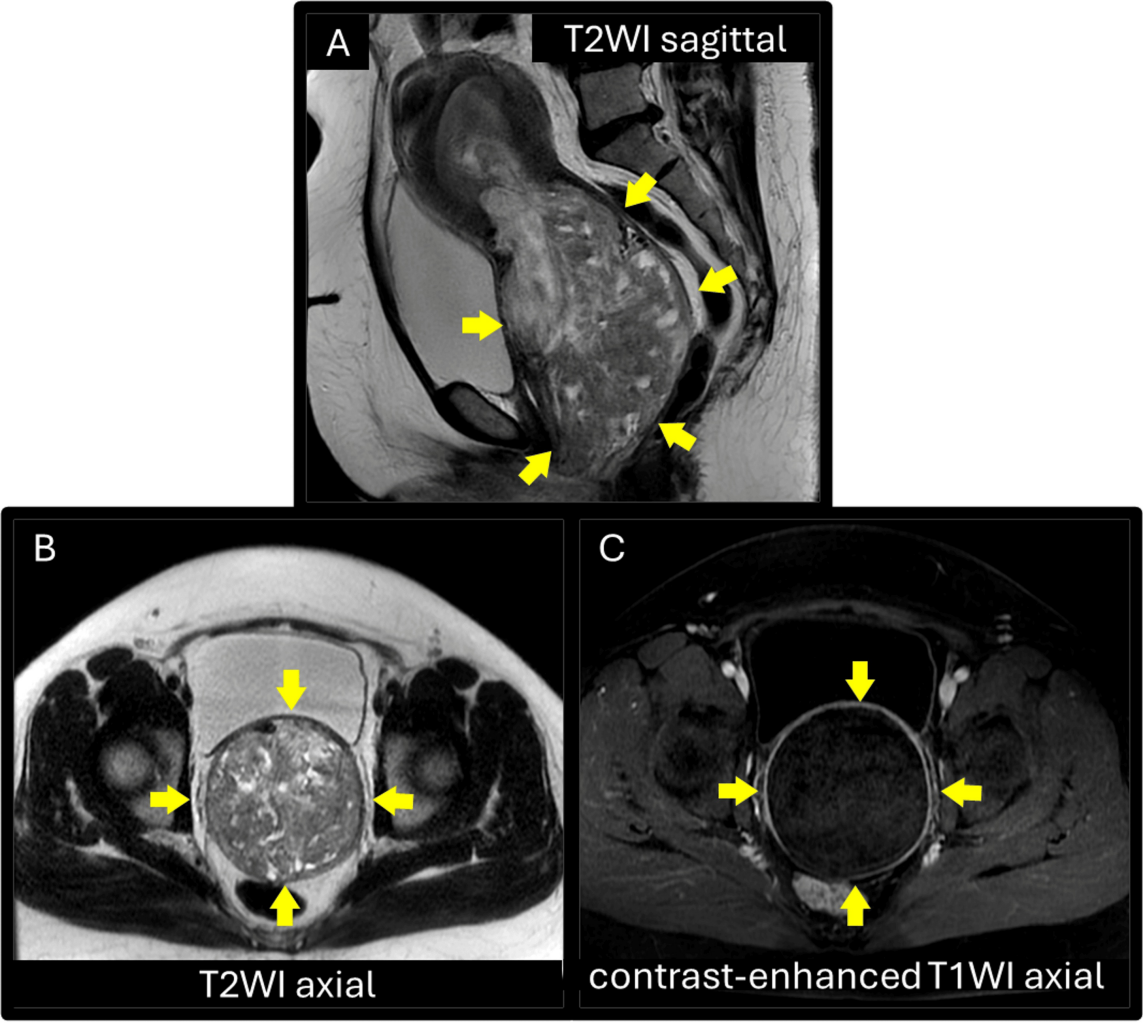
Pelvic magnetic resonance imaging of the patient at her first presentation prior to emergency hysteroscopic surgery. (A) T2-weighted mid-sagittal image showing the tumour with a heterogeneous signal arising from the uterine fundus and prolapsing into the vagina. (B) T2-weighted axial image and (C) contrast-enhanced T1-weighted axial image at the level of maximal diameter of the mass showing no contrast enhancement, indicating necrosis of the tumour. T2WI, T2-weighted image; T1W1, T1-weighted image.
